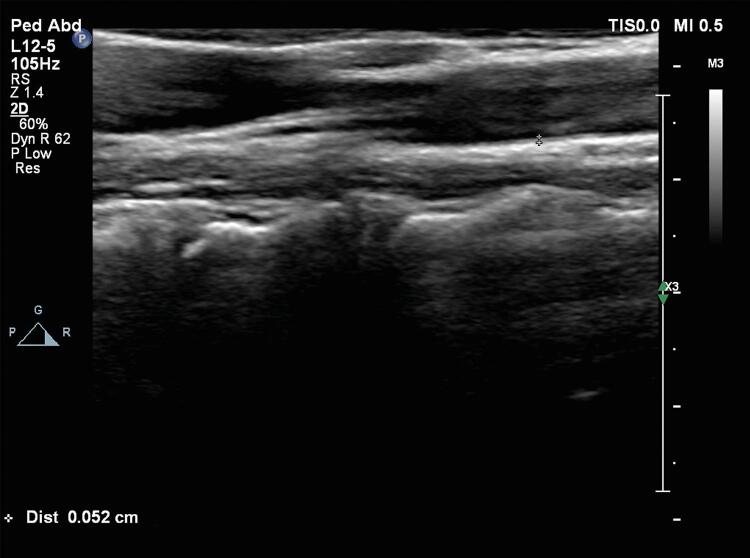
Medida da espessura médio-intimal da artéria carótida comum por ultrassonografia modo B em um paciente com talassemia menor (valor normal: 0,57 mm).
